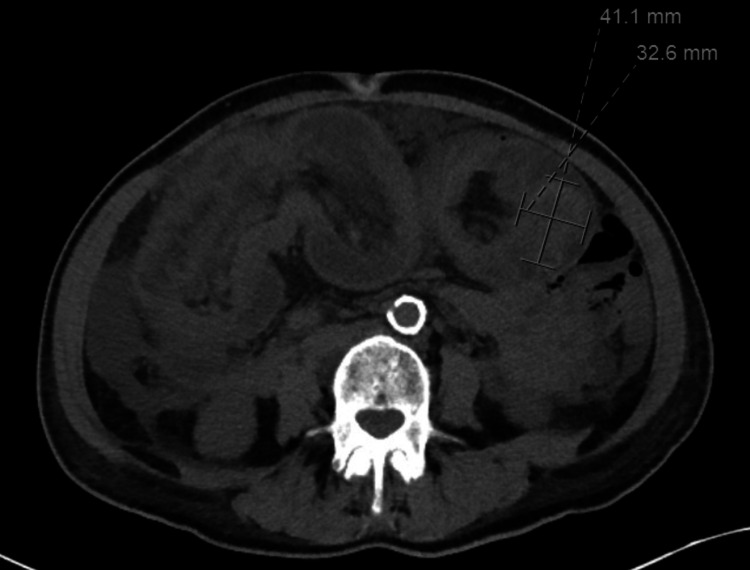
Axial slice of CT of the abdomen/pelvis demonstrating a large colonic intussusception in the mid-abdomen with extensive wall thickening and edema. Shown also is possible lead point at the distal portion of the invaginated bowl concerning for neoplasm.
